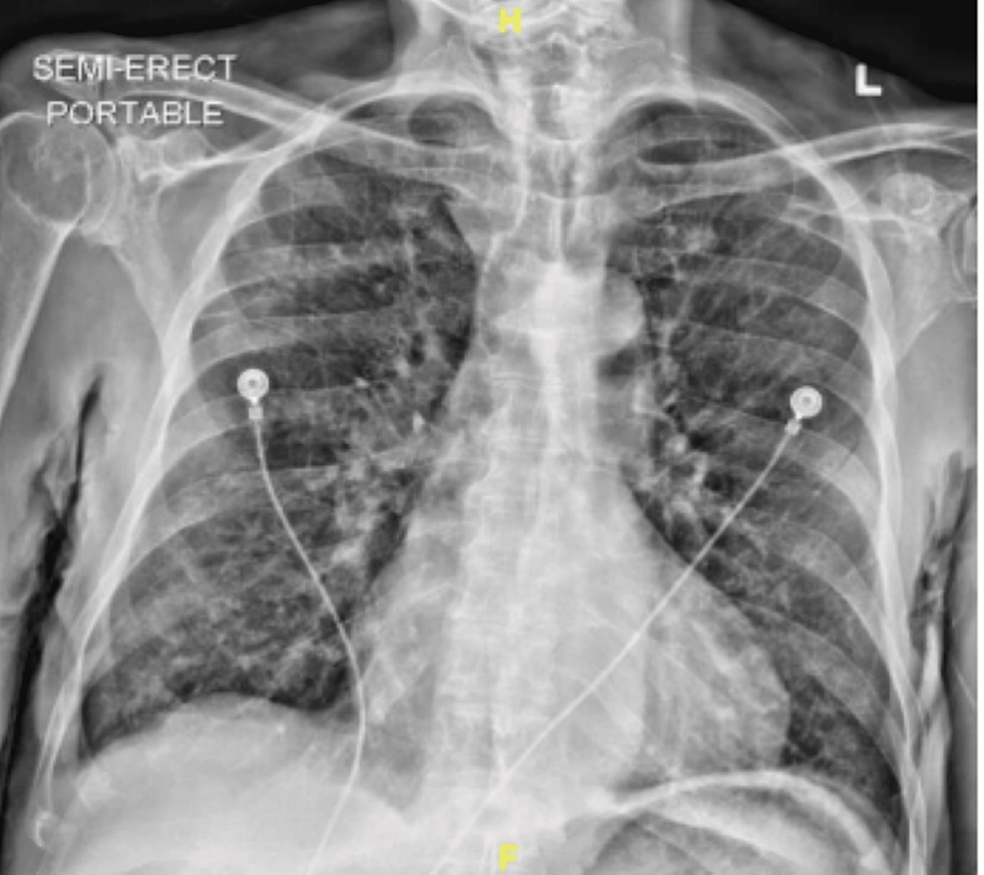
Portable chest x-ray at the time of admission demonstrating no evidence of focal consolidation, pleural effusion, or identifiable acute pulmonary pathology
